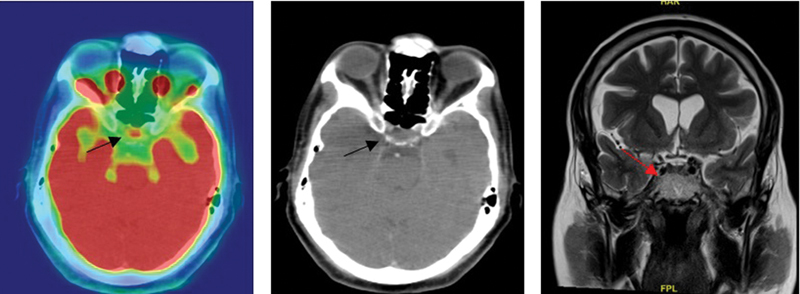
A 52-year-old male with disseminated tuberculosis—pituitary involvement (standardized uptake value [SUV] 5.5). Magnetic resonance imaging (MRI) brain showed enlarged pituitary gland with enhancing sellar surpraseller lesion involving the pituitary stalk. Follow-up MRI showed resolution of the lesion.
